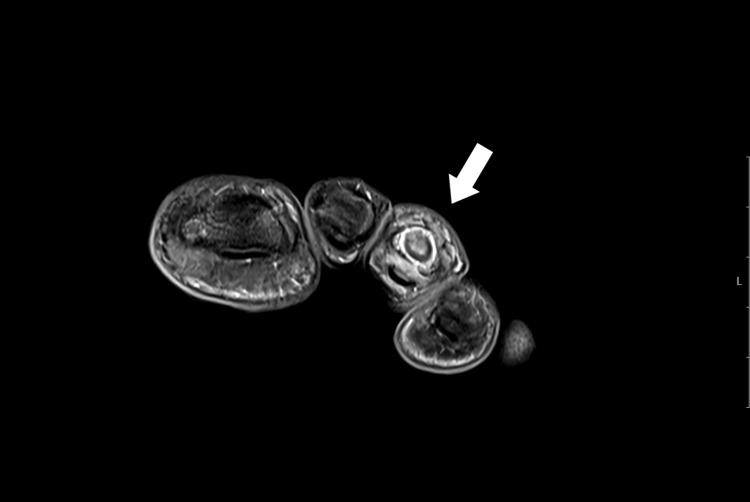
Coronal T2-weighted MRI showing a central high-intensity signal in the middle phalanx of the third toe, with a thin surrounding area of edema
